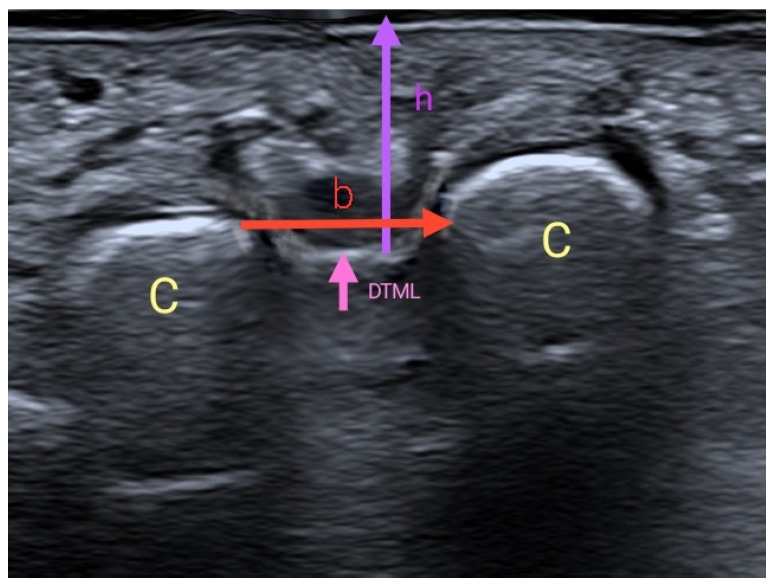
Ultrasound imaging of the measurements. C: Metatarsal heads 3 and 4 (M3 and M4). DTML: Deep transverse metatarsal ligament. h: Height: Distance between the DTML and the plantar skin in the middle area between M3 and M4. b: Base: Distance between M3 and M4.
